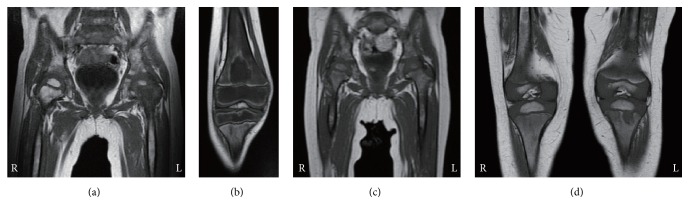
MRI findings after the remission induction chemotherapy. Bone marrow in all cases contains increased signal intensity on T1-weighted images. (a) Case  1, (b) case 2, (c) case 3, and (d) case 4.
